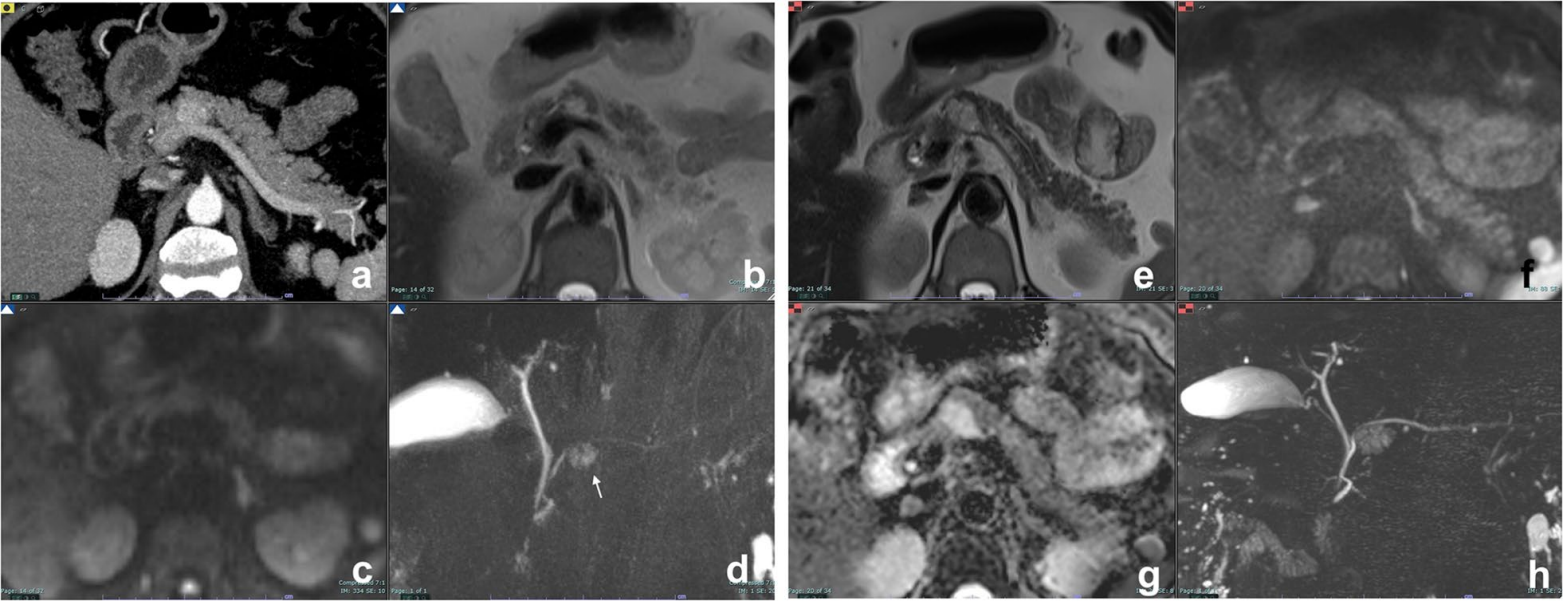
Serous cystadenoma, pseudosolid pattern. A small hypervascular lesion in the body of the pancreas is visible at contrast-enhanced CT, arterial phase (a). On T2w, the lesion is hyperintense (b), with no high signal intensity at DWI b 800 (c). At MRCP (d), the cystic components are well recognizable (arrow) and MPD is normal. e–h Follow-up at 5 years. A slight increase in size can be appreciable with no others significant changes. At ADC map (g), the lesion is clearly hyperintense due to cystic content
